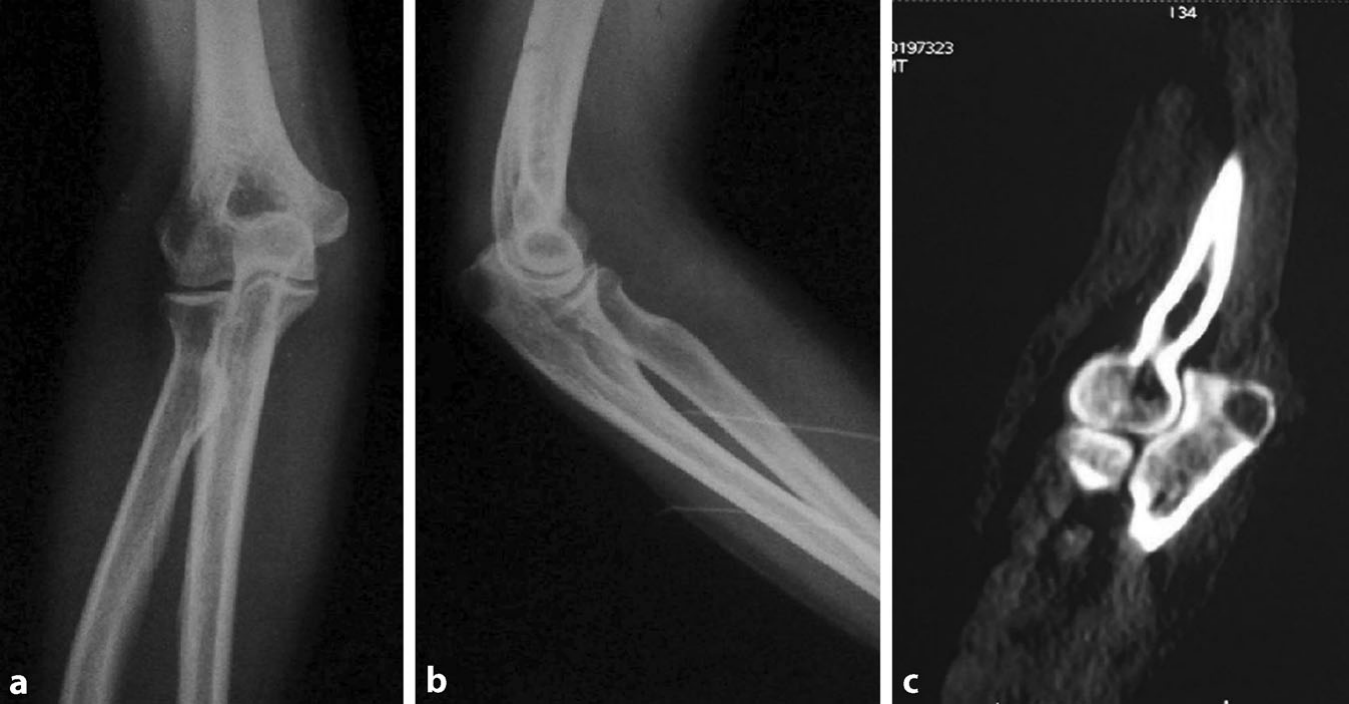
a Anteroposterior plain radiograph of the right elbow indicated that there was a osteolytic lesion in the upper back end of olecranon. b Lateral plain radiograph showed that there was a poorly defined osteolytic lesion in the upper back end of olecranon. c Computed tomography scan revealed a poorly defined osteolytic lesion in the upper back end of olecranon
